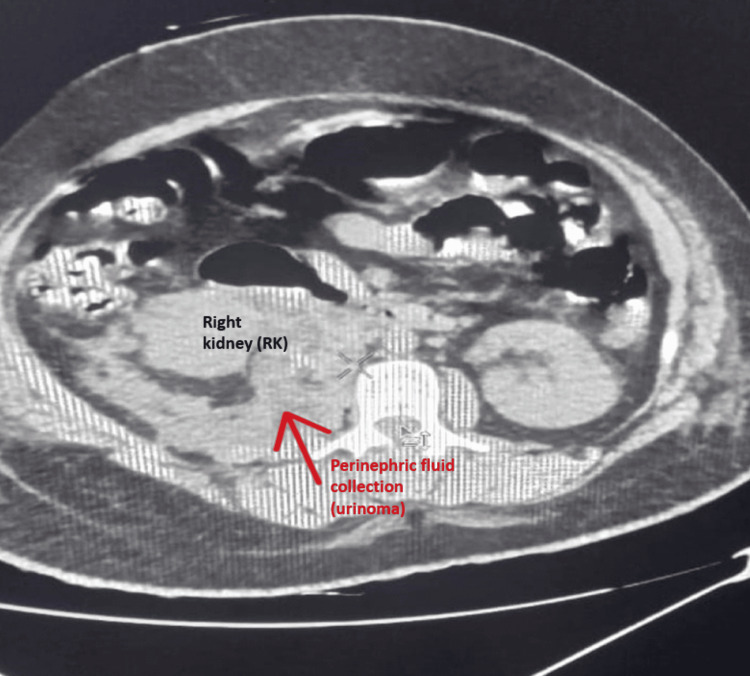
Axial CT abdomen demonstrating right perinephric fluid collection Axial noncontrast CT abdomen at the level of the renal hilum demonstrating a right-sided perinephric fluid collection (arrow) in the setting of obstructive uropathy secondary to ureteric calculi. The collection is situated within Gerota's fascia and is consistent with urinary extravasation forming a urinoma. The right kidney is visible to the right with mild hydroureteronephrosis CT: computed tomography
